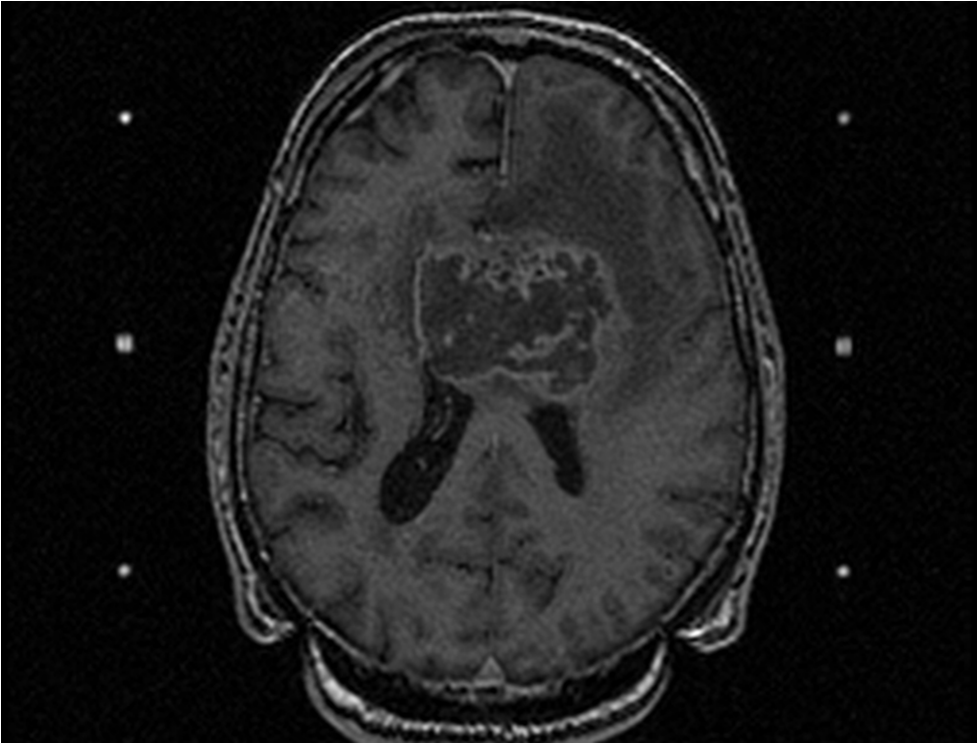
MRI performed for a seventy-three-year-old man presented to the emergency department with bilateral muscular weakness members, cognitive disorders and KPS of 60. The T1W sequence with gadolinium injection showed a large bifrontal GBM invading the corpus callosum. Stereotactic biopsy revealed the presence of a glioblastoma multiforme (confirmed by independent reviewing). This patient had almost all factors of poor prognosis (sex, age, low KPS, tumor localization, no surgical treatment) but received concomittant radiochemotherapy with temozolomide and survived 4 years.
